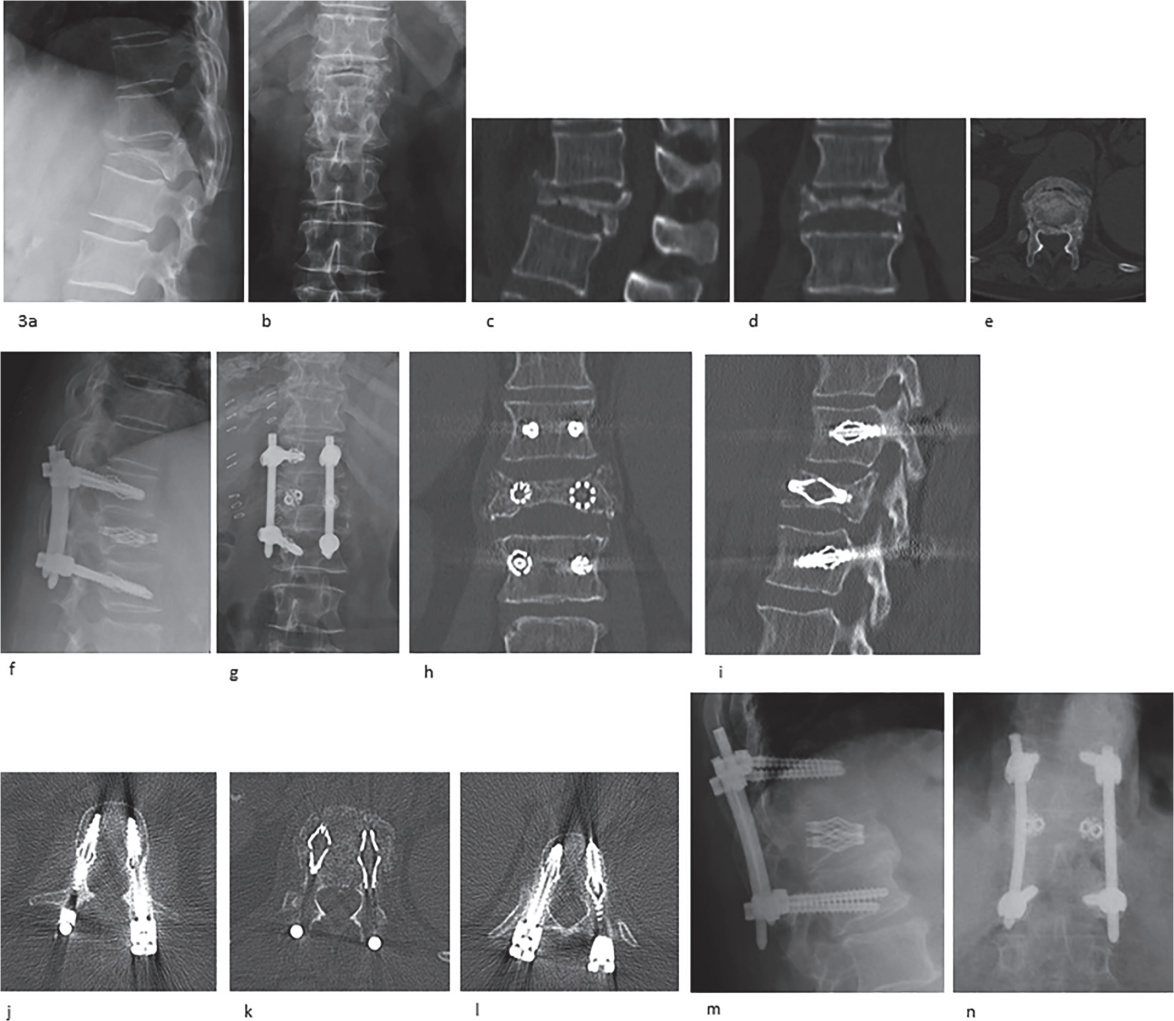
Radiographs pre- (a-e), post-surgery (f-l) and for final follow-up (m,n) showing a T1 burst fracture and operative treatment by an internal fixateur one level above and below the fractured vertebra body and reduction by transpedicular placement of two titanium mesh cages, all via minimum invasive technique.
